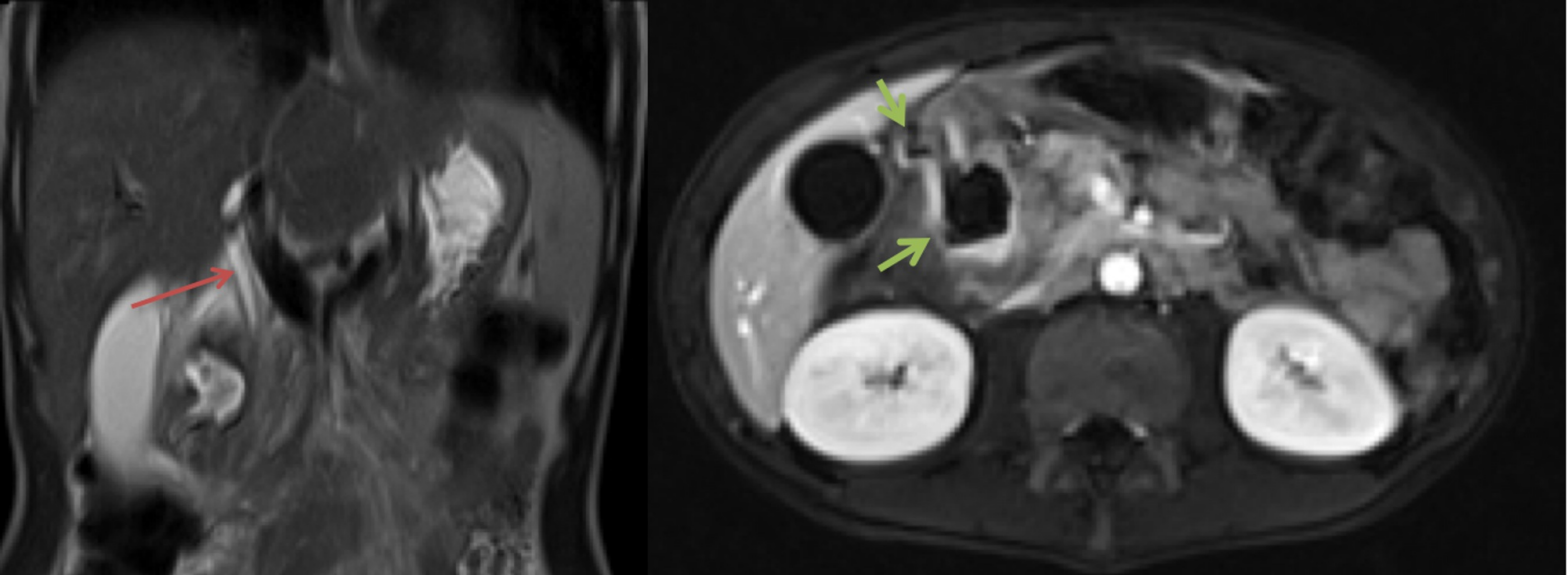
MRI selected images: Coronal T2WI (a) and axial T1WI (b) with fat sat and post IV contrast administration demonstrate the duodenal diverticula (green arrows) with air fluid level. The MR images confirm the diverticulitis changes and exclude the pancreatic pathologies. Note the distended CBD (red arrow).
